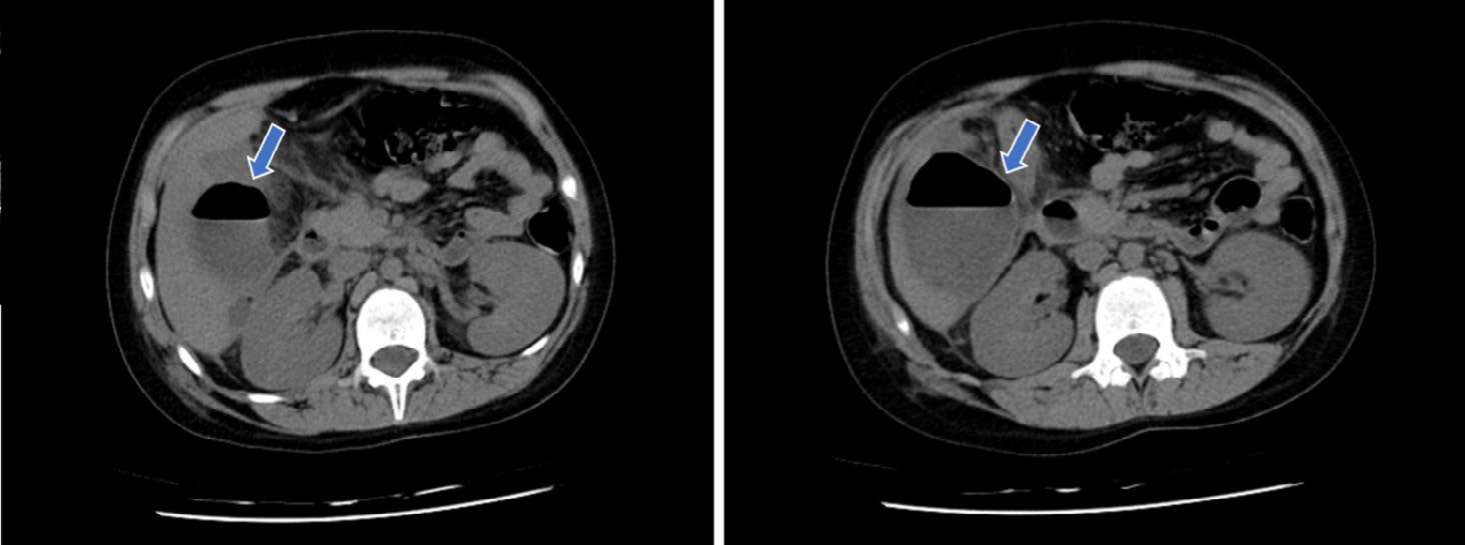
Axial abdominal CT scan images of the patient. The arrows indicate the hepatic abscess.
